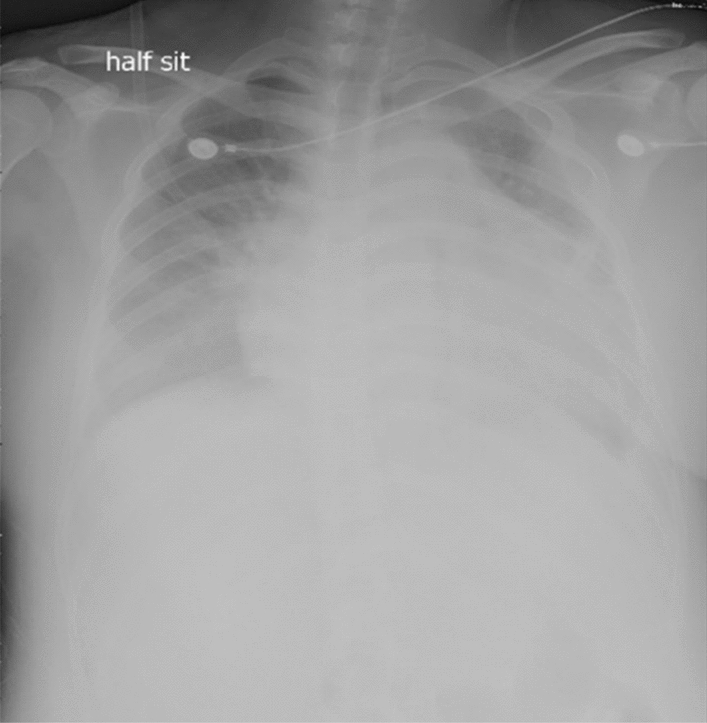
Postoperative chest X-ray from the ICU
